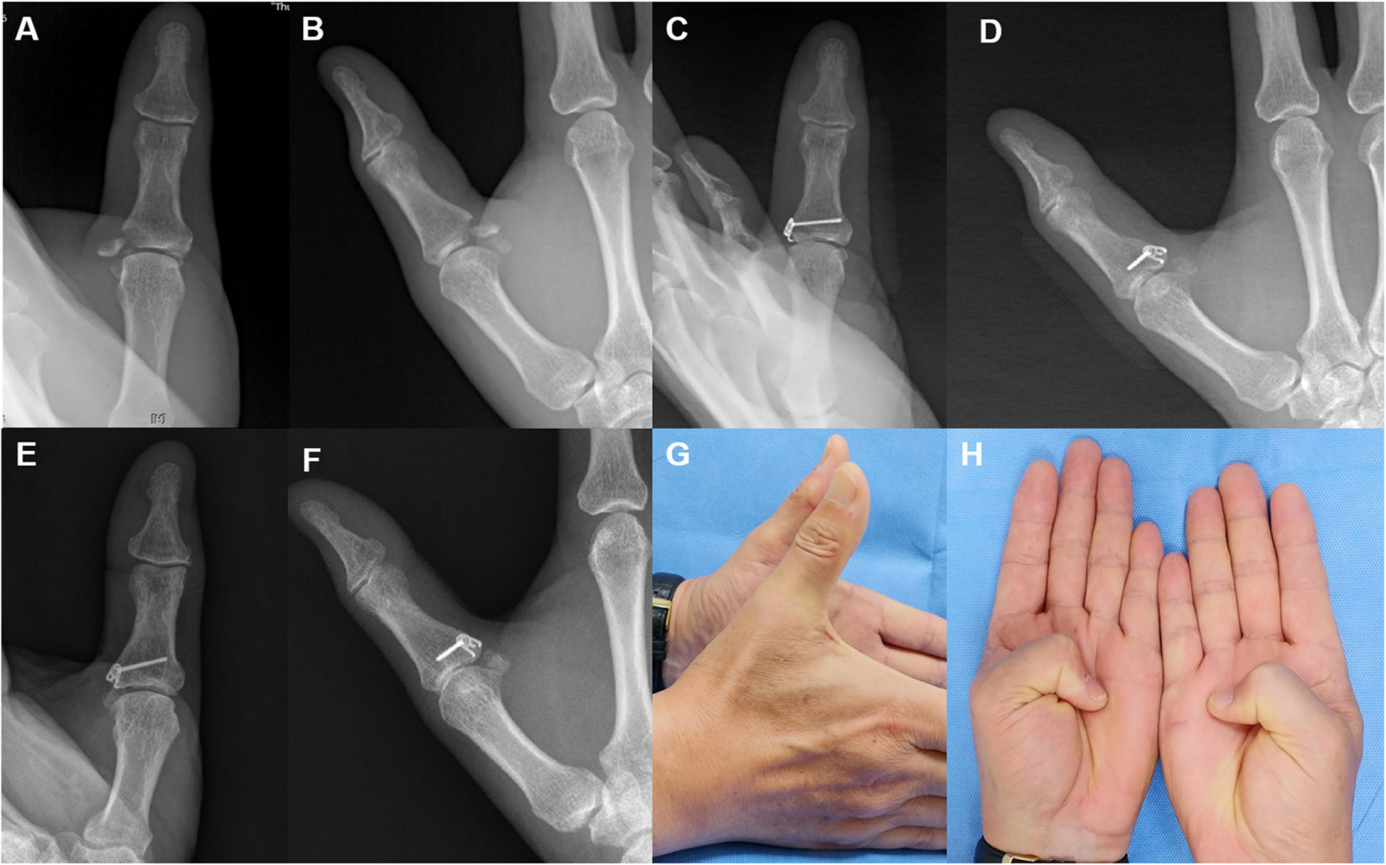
Ulnar collateral ligament avulsion fracture (a, b). The avulsed fragment was reduced and captured by the mini-hook plate (c, d). Plain radiography 55 months post-operation revealing that the osseous fragment was well united (e, f). The total range of MP joint motion was 82%, and the pinching strength was 98% compared to the values of the same contralateral joint (g, h)
